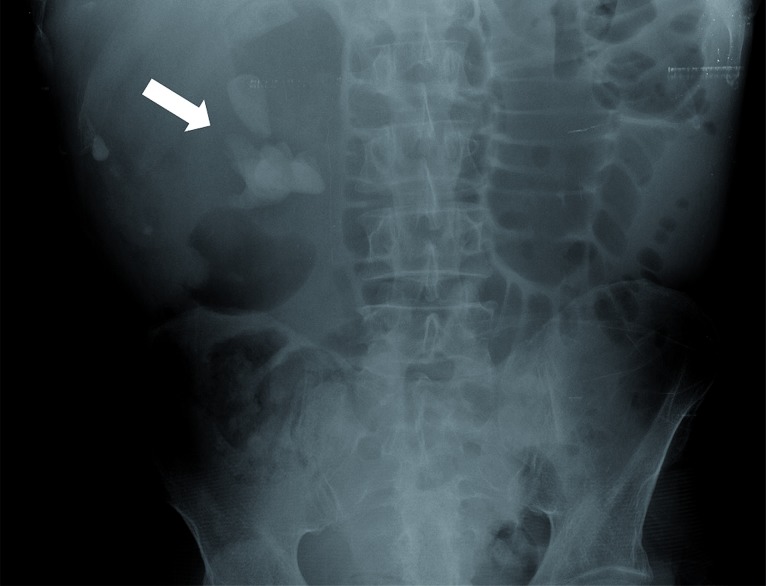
Abdominal radiography demonstrates staghorn calculus in right kidney.
